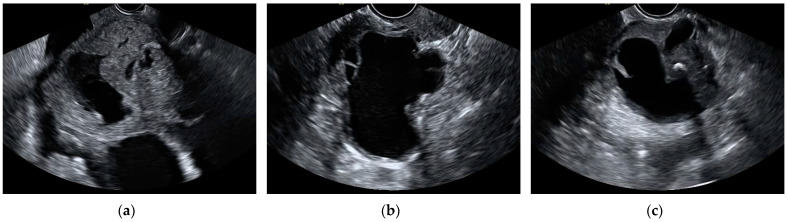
Still ultrasound images of malignant ovarian masses misclassified as benign by the aggregate model. (a) Endometrioid ovarian carcinoma, (b) high-grade serous ovarian carcinoma, and (c) high-grade serous ovarian carcinoma.
